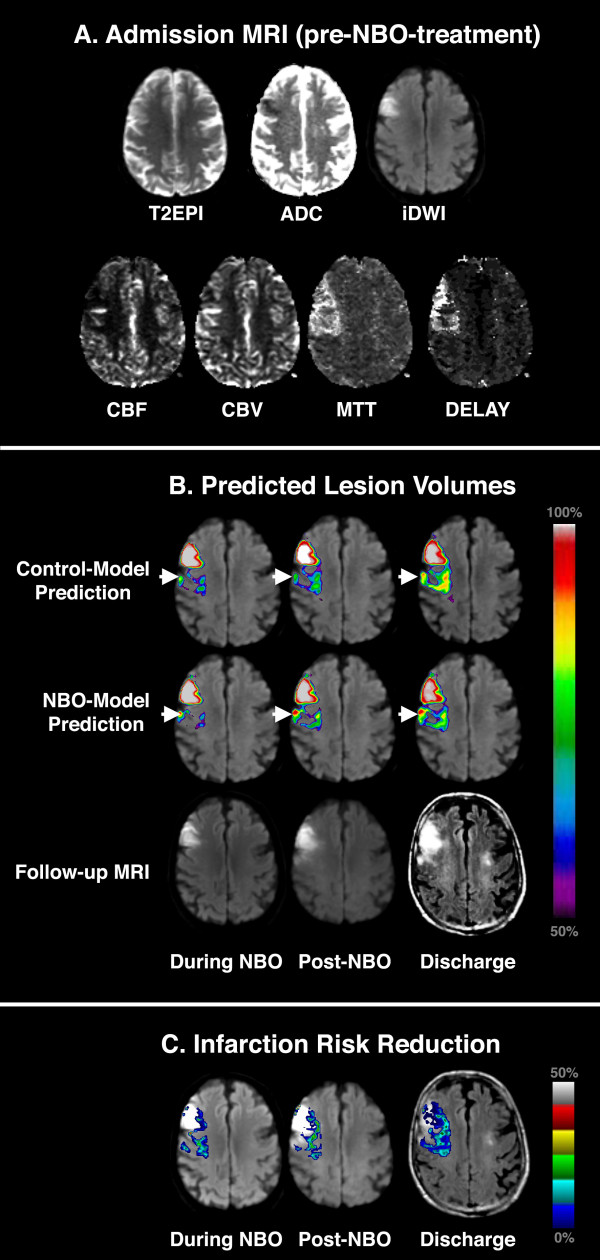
Example 2 of Predicted Lesion Volume Development. (A) Admission MRI dataset for a 64 year-old male patient imaged at 4.5 hours after stroke symptom onset who was treated with NBO. (B) Corresponding GLM-predicted lesion risk maps for Control and NBO-models at each subsequent time point of imaging, and (C) Overlay map of differences between the two models showing ischemic tissue that is 'potentially salvageable' with NBO therapy. The GLM-predicted lesion volumes are a synthesis of data from the admission MRI only. In this patient, the risk of tissue infarction in DWI/PWI mismatch regions was predicted to increase over time. For clarity, only GLM-predicted lesion risk > 50% are shown overlaid on acute DWI. Note that the amounts of tissue predicted to infarct at all time-points with the Control-models were greater than their NBO-model counterparts, with difference principally in the DWI/PWI mismatch region (arrowheads). In the difference maps (C), the color scale represents infarction risk reduction as a result of NBO-therapy (conversely, larger values represent greater likelihood of infarction if the patient was given Control-treatment).
